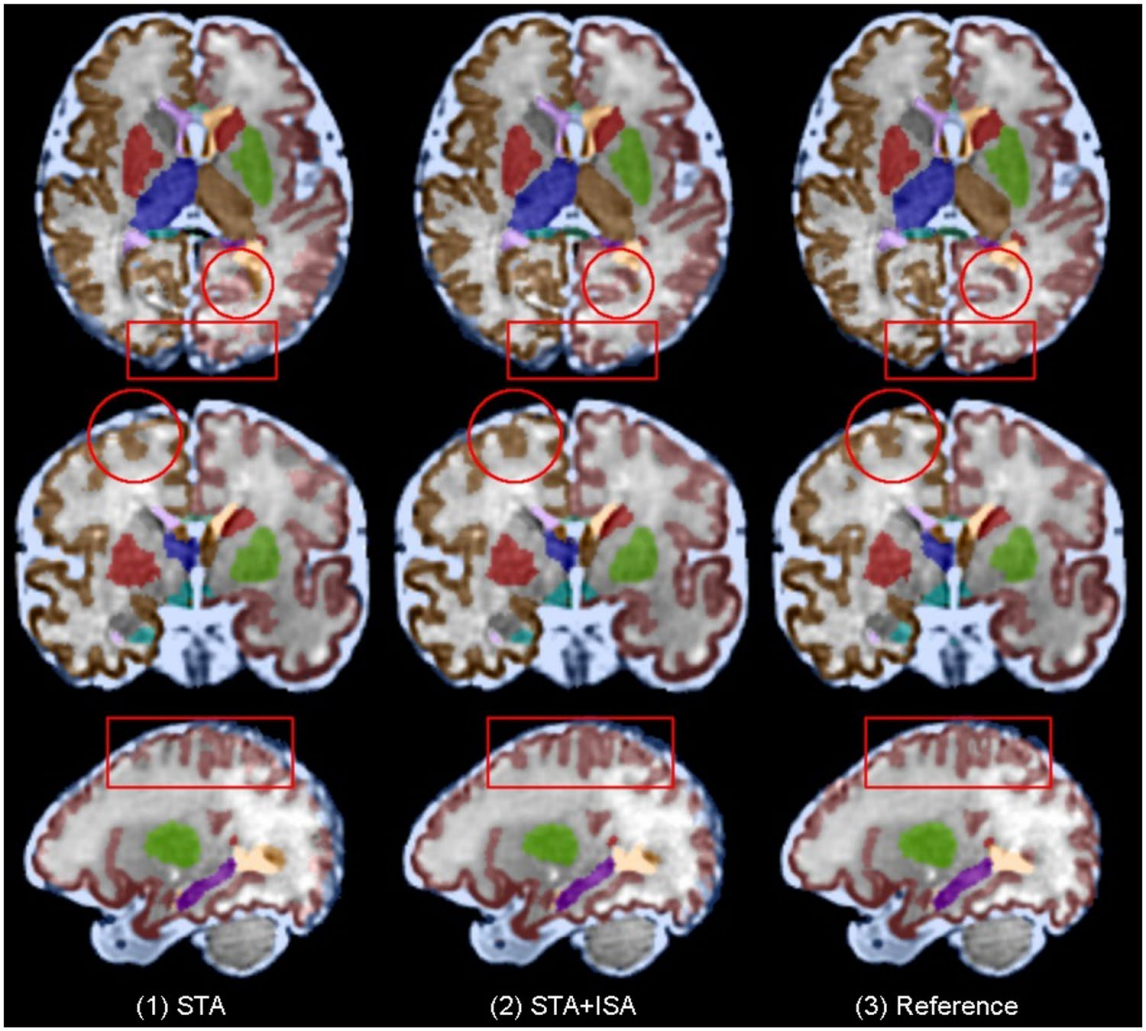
Visual assessment and comparison of atlas-based segmentation of fetal brain MRI using multiple atlases: (1) segmentation using the spatiotemporal fetal brain MRI atlas (STA), (2) segmentation using the combination of the spatiotemporal atlas and individual-subject atlases (STA + ISA), and (3) reference standard. The circles and squares point at some of the areas in which the methods performed differently. Overall the results were satisfactory. As expected due to the use of a larger number of atlases, slightly better performance was observed for STA + ISA. For quantitative comparison of methods (STA and STA + ISA) we relied on the analysis of average DSC metrics reported in Table 1. Labels that are visible on these images are CSF, corpus callosum, developing white matter, and left and right cortical plates, ventricles, thalami, hippocampi, amygdalae, and caudate nuclei.
